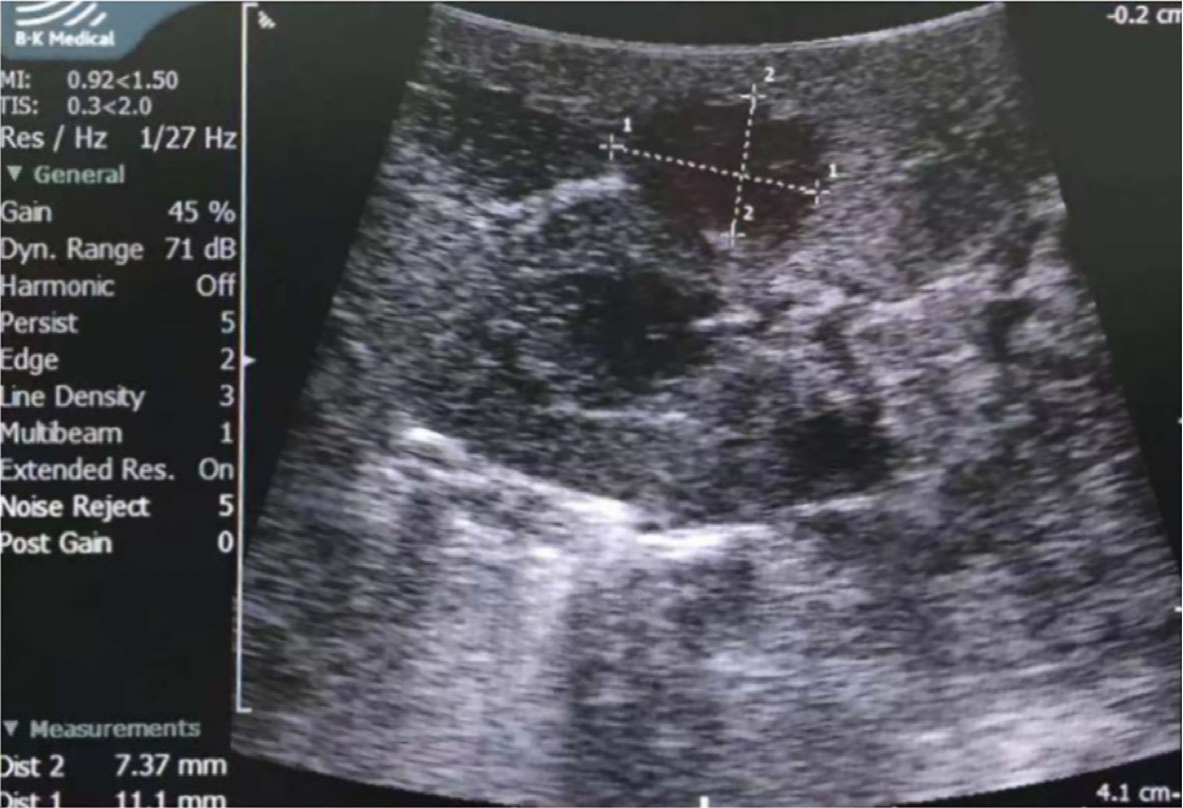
Laparoscopic ultrasound of Patient No. 8. The tumor was hypoechoic and was measured 7.4×11.1mm by ultrasound.
